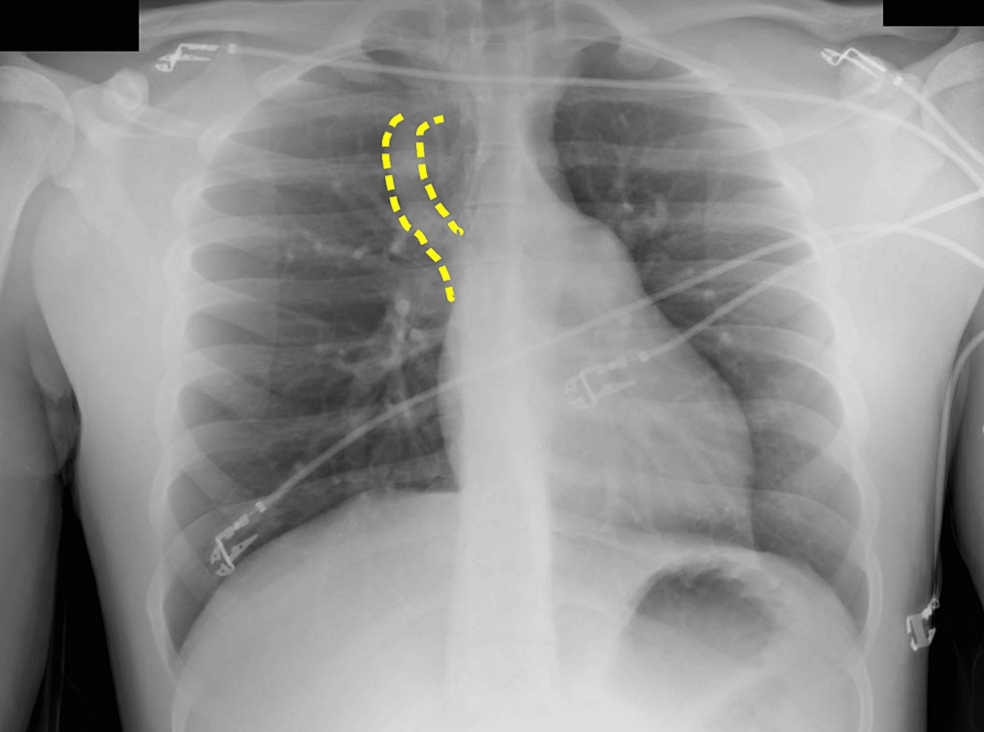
Posterior anterior (PA) chest X-ray Aorta with normal course and density. Image suggesting prominence of the azygos vein (yellow intermittent line). Both pulmonary hilia without evidence of lesions. The cardiac silhouette preserves shape, size, and contours.
